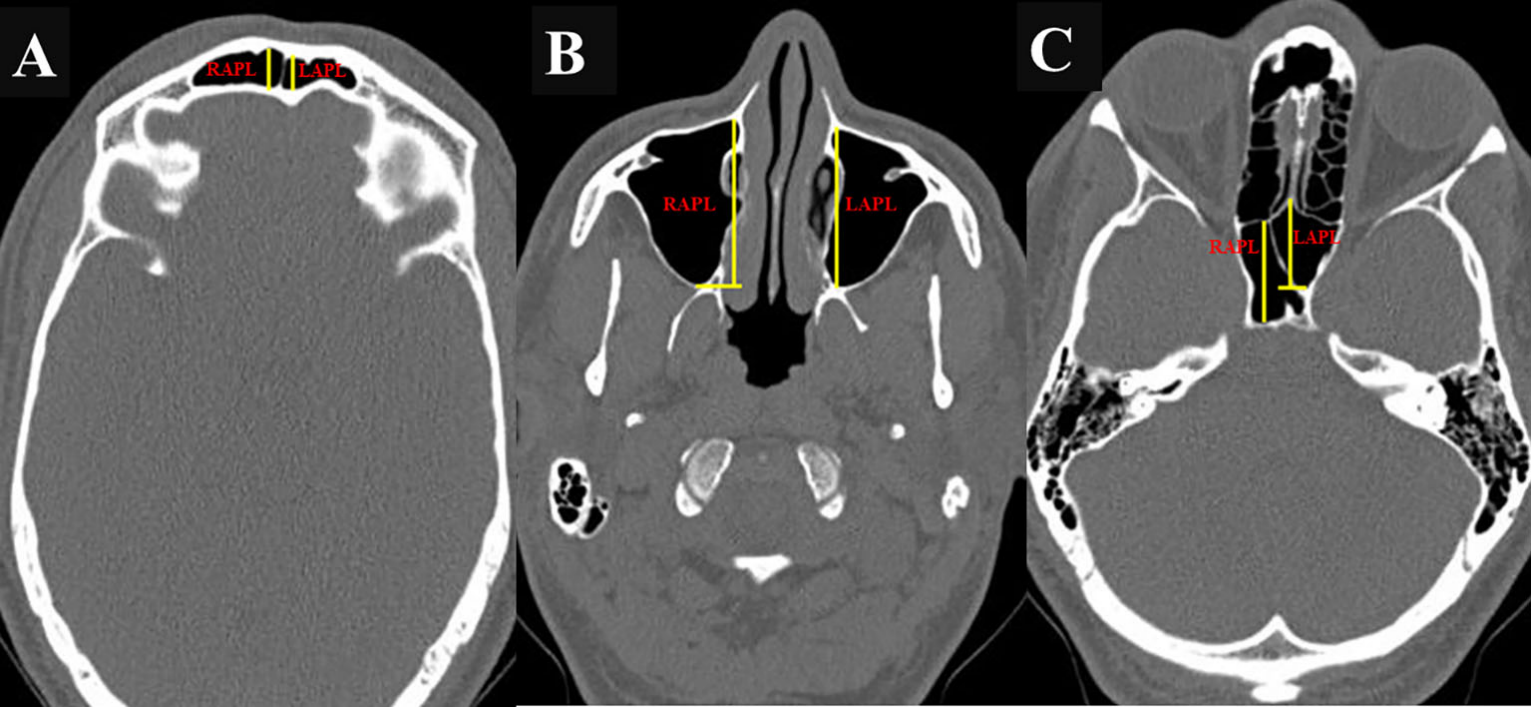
(A,B,C): Axial CT image showing the AP measurement of FS, MS and SS respectively. (RAPL- right anteroposterior length; LAPL- left anteroposterior length).
